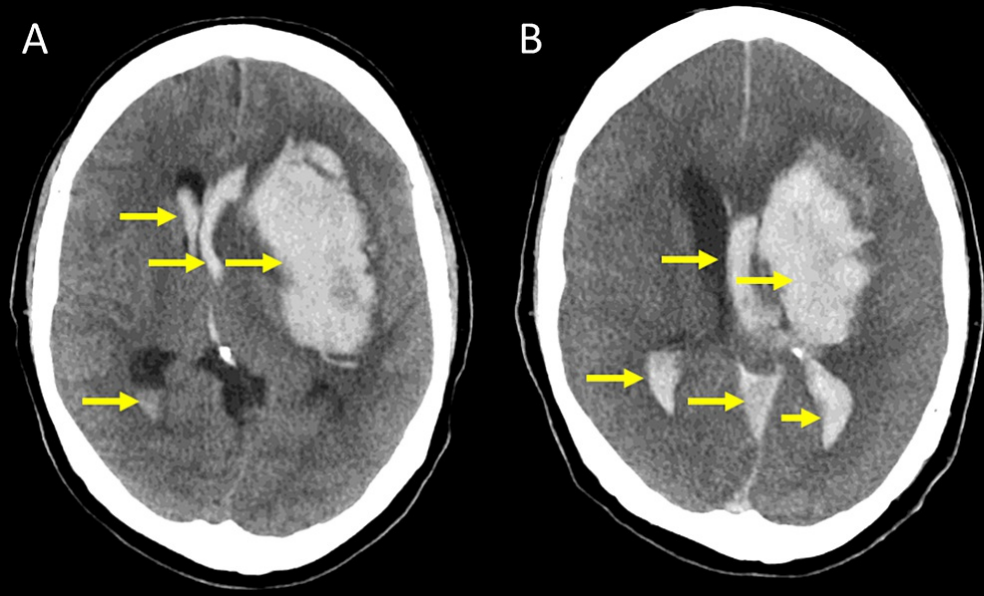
(A) Initial axial computed tomography (CT) scan taken after the presentation to the emergency department. (B) A follow-up axial CT scan of the head taken five days later demonstrates minute differences, even after medical management.
